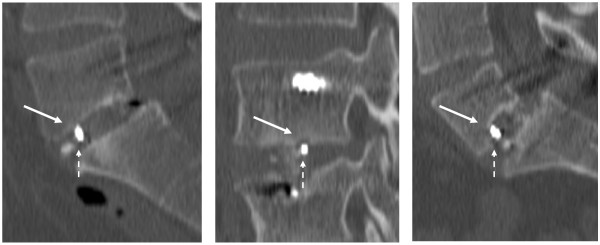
Vertebral subsidence. Examples of no (left), moderate (middle), and pronounced (right) vertebral subsidence, as assessed on CT by the degree of sinking of the intervertebral cage into the vertebral body (closed arrow) and the position of the radiological markers of the intervertebral cage (open arrow).
